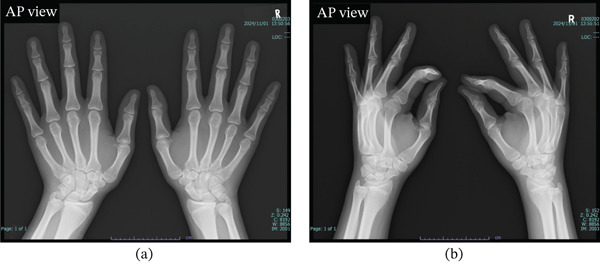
Initial radiographs of both wrists. (a, b) Plain x‐ray images of both wrist joints at the initial visit to our department. No findings suggestive of rickets, such as cupping or flaring, were observed (AP view).
